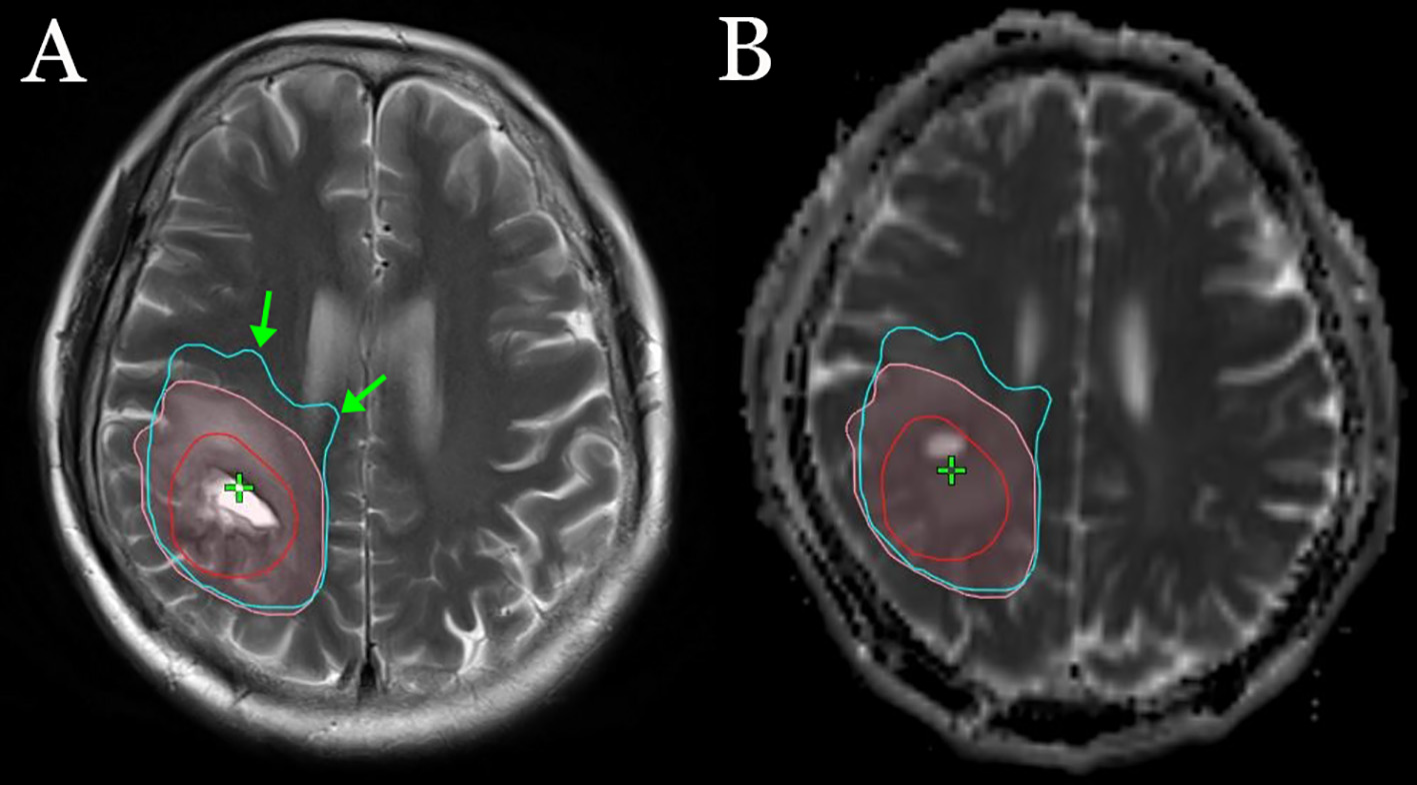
An example of the sites of tumor infiltration shown by T2 (A) and the DTI-p map (B). The red line represents GTV, the pink lines represent the sites of tumor infiltration shown by T2, and the light blue lines represent the sites of tumor infiltration shown by the DTI-p map. The green arrow represents the depth of tumor infiltration shown by the DTI-p map was deeper than T2/FLAIR in the direction of adjacent main nerve fiber bundles.
